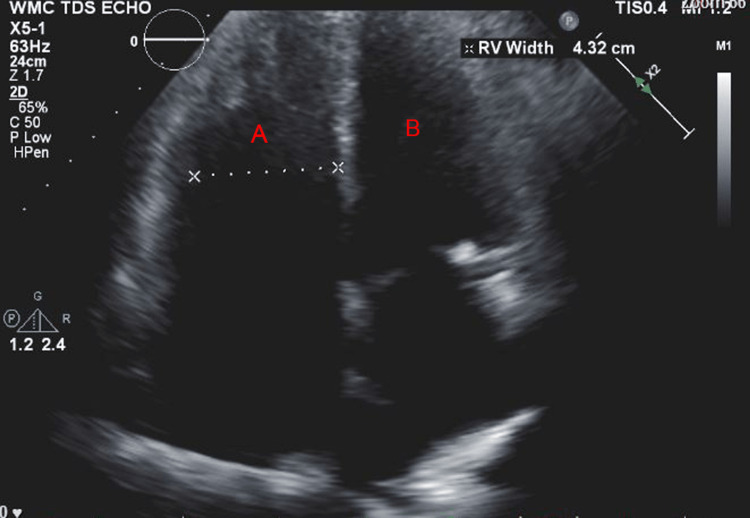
Preoperative TTE apical four-chamber view showing (A) dilated right ventricle with increased wall thickness and (B) left ventricle TTE: transthoracic echocardiogram
